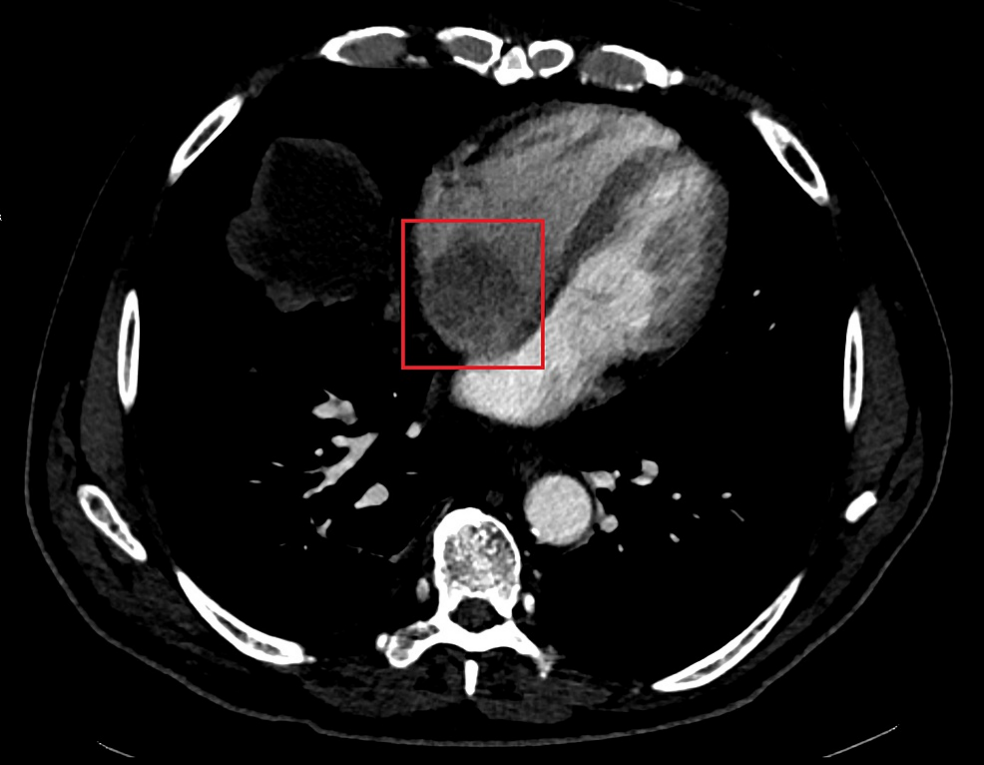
Abdominal CT scan showing the right atrium with thrombus (red box).
